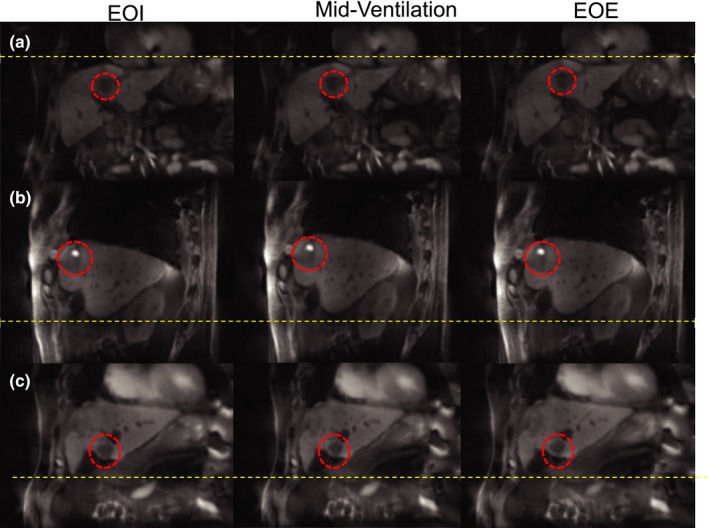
Example SS‐4D‐MRI images of respiratory phases at end of inspiration (EOI), mid‐ventilation, and end of expiration (EOE) in a liver patient with coronal (a,c) and sagittal (b) views of different tumor lesions. The red circles represent tumor location.
